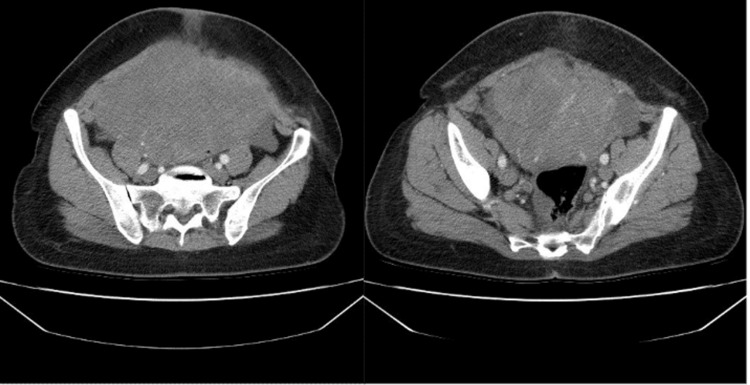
CT scan demonstrating a 12 cm pelvic mass in the right adnexal region with irregular borders, central necrosis, and displacement of adjacent digestive structures. CT, computed tomography
